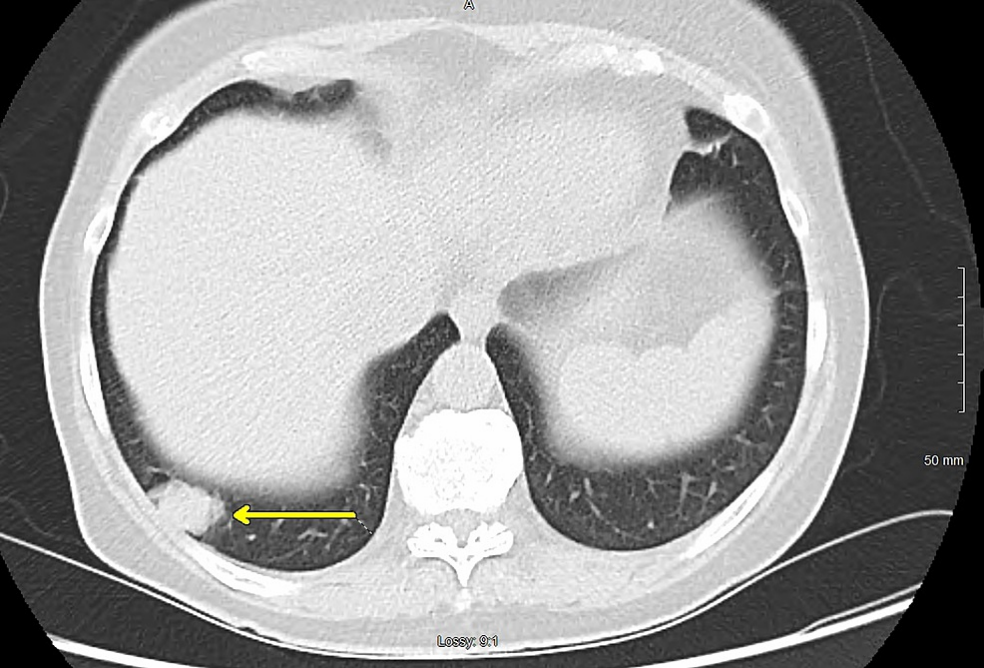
CT scan in the axial view demonstrating a non-calcified, lobulated mass (arrow) in the right costophrenic angle CT: computed tomography
